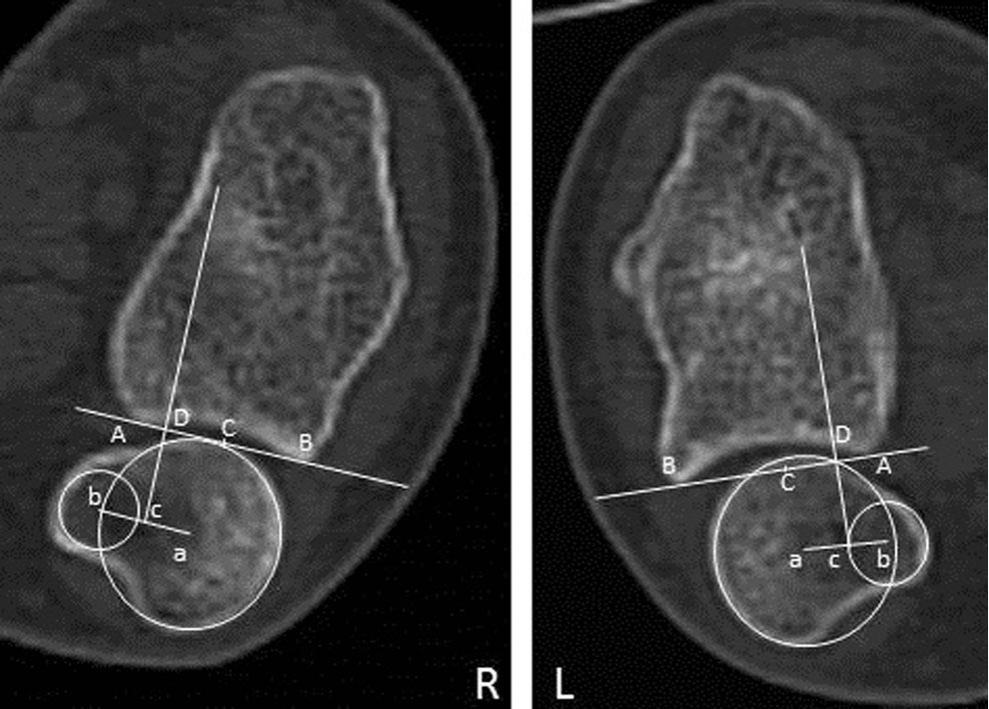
The CT scan in pronation of a 24-year-old female, 4 years and 4 months after a complete intraarticular fracture on the left side. Using the epicenter method, this wrist was measured out of the normal range, compared to normal values based on mean measurements. She indicated no (0) pain using the VAS score
